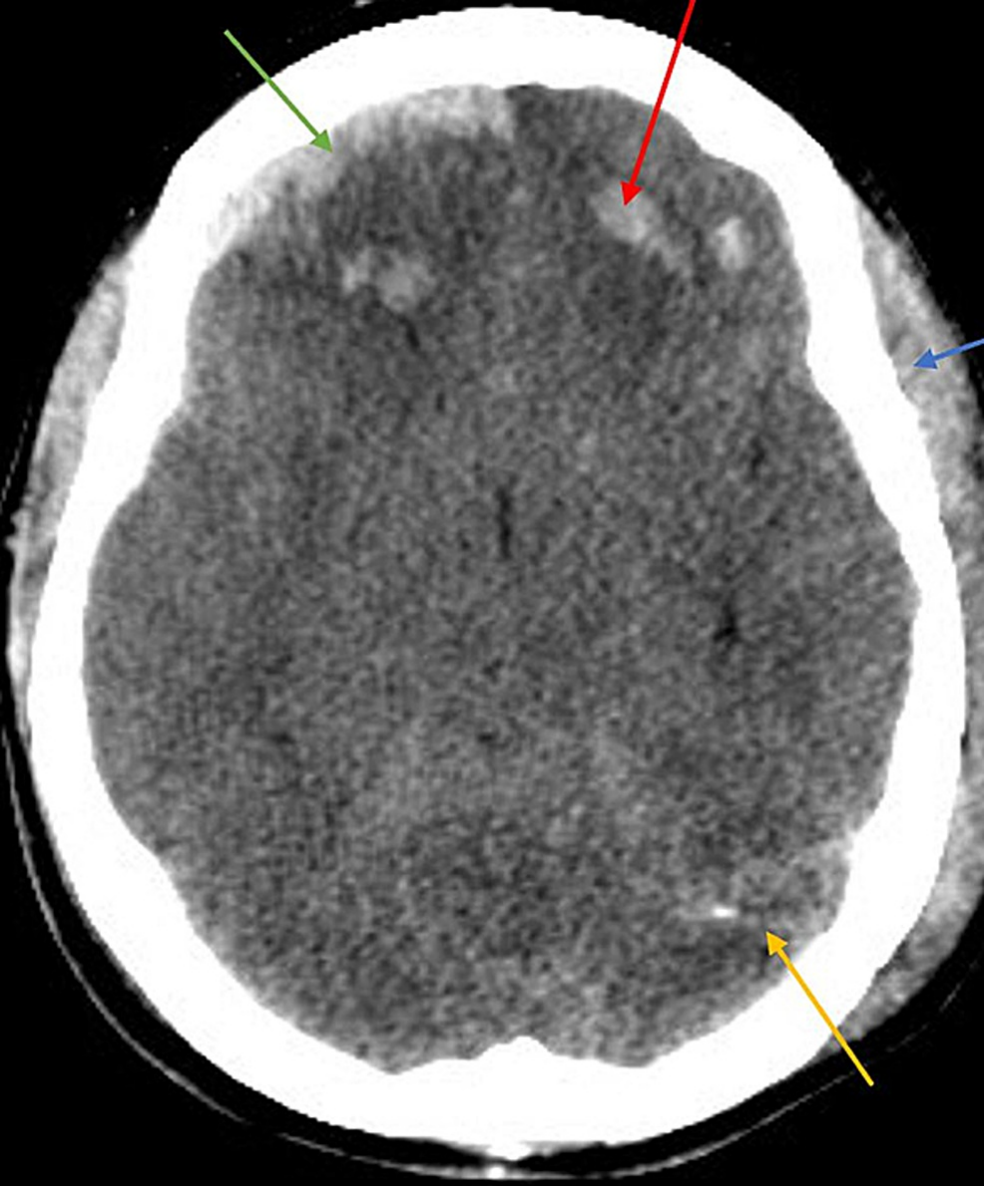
CT head without contrast on admission Green arrow: traumatic right frontal sub-dural hematoma, red arrow: traumatic left frontal hemorrhagic contusion, blue arrow: scalp hematoma; yellow arrow: traumatic sub-arachnoid hemorrhage
